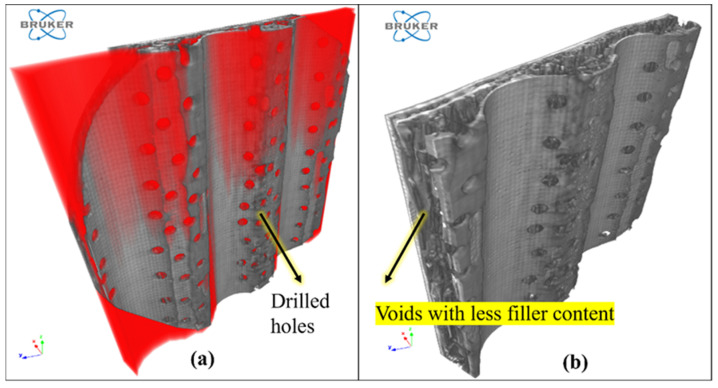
CT-scanned image of RCR-Perforated panel.
